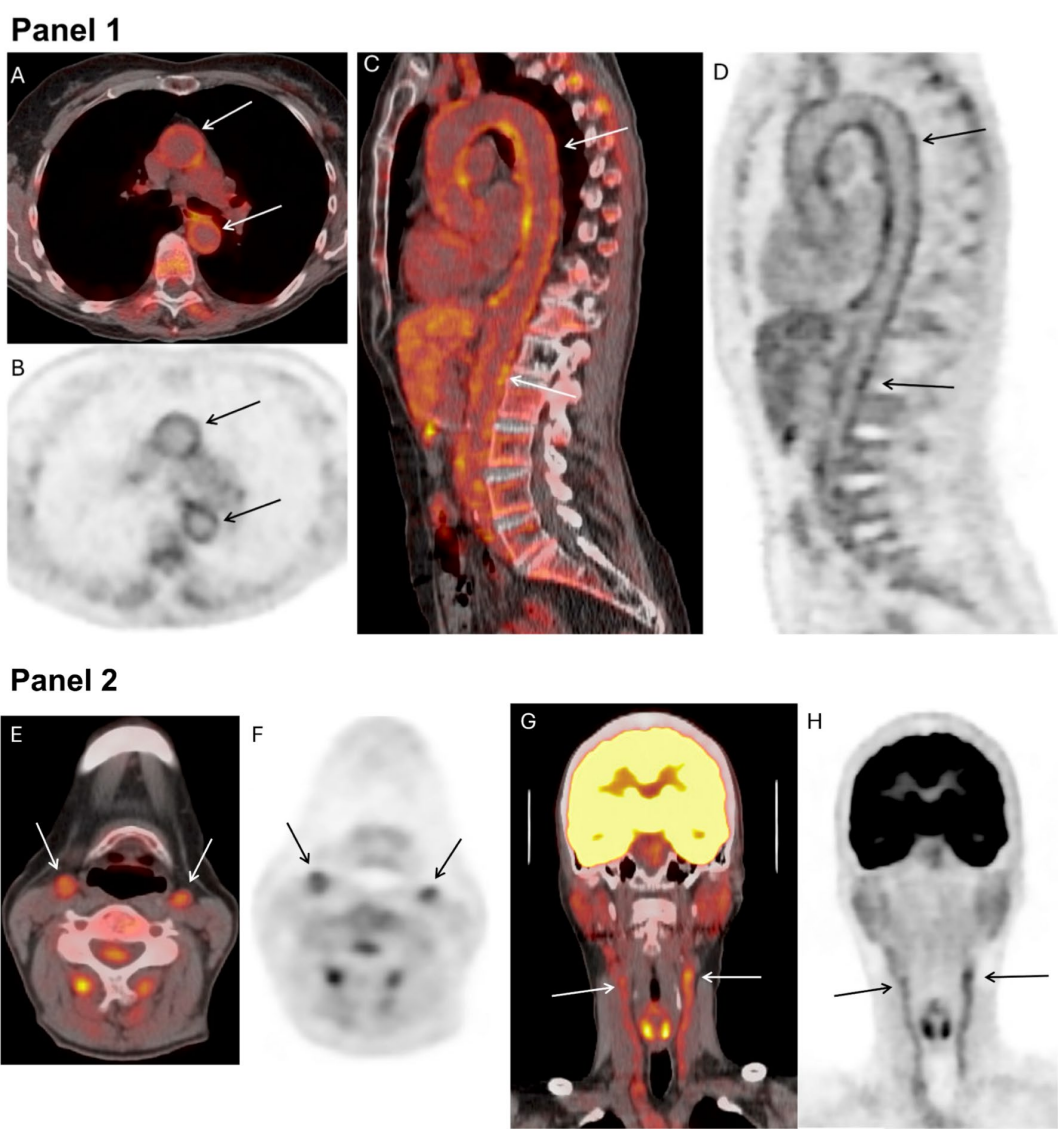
Active large vessel vasculitis. A 63-year-old female with a recent diagnosis of giant cell arteritis had an ^18^F-fluorodeoxyglucose (^18^F-FDG) PET/CT performed to assess for extent/activity of disease elsewhere. A Vasculitis FDG PET/CT was performed from vertex of the skull through the mid thighs, with a dedicated head and neck bed acquisition. Panel 1: Fusion and PET images are displayed, images A and B correspond to the axial plane at the level of ascending and descending aorta, while images C and D are oriented to the thoracoabdominal aorta. There is concentric FDG uptake along all the segments of the aorta (Images A-D, *white arrows* on fusion images and *black arrows* on PET images), consistent with active vasculitis. Panel 2: Fusion and PET images of the head and neck are displayed, images E and F correspond to the axial plane at the level of common carotid arteries, while images G and H correspond to the coronal plane. There is abnormal FDG uptake along the common carotid arteries (Images E-H, *white arrows* on fusion images and *black arrows* on PET images), consistent with active vasculitis of the common carotid arteries in the context of known giant cell arteritis
